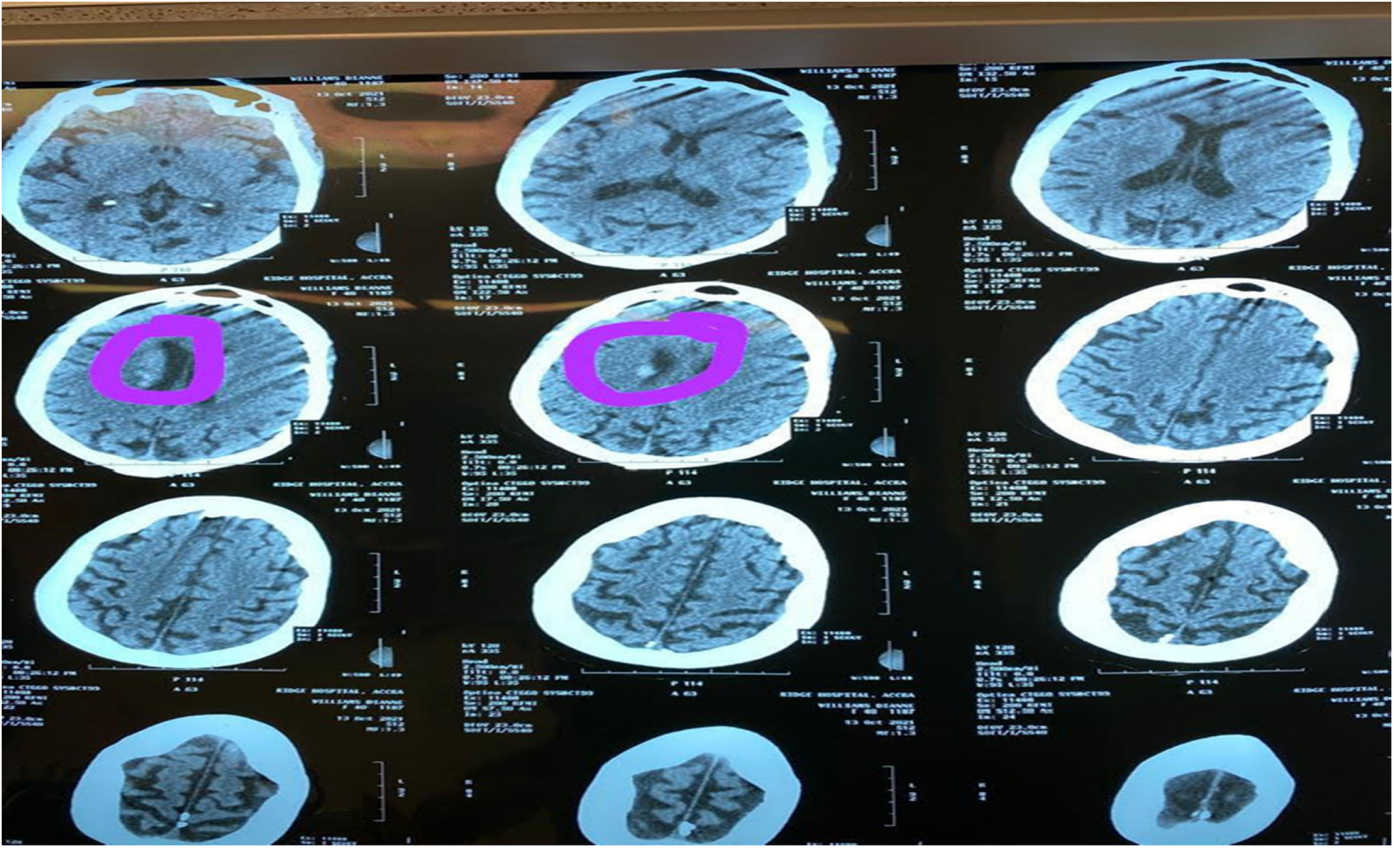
CT scan of the head taken during recurrent episodes of seizures
